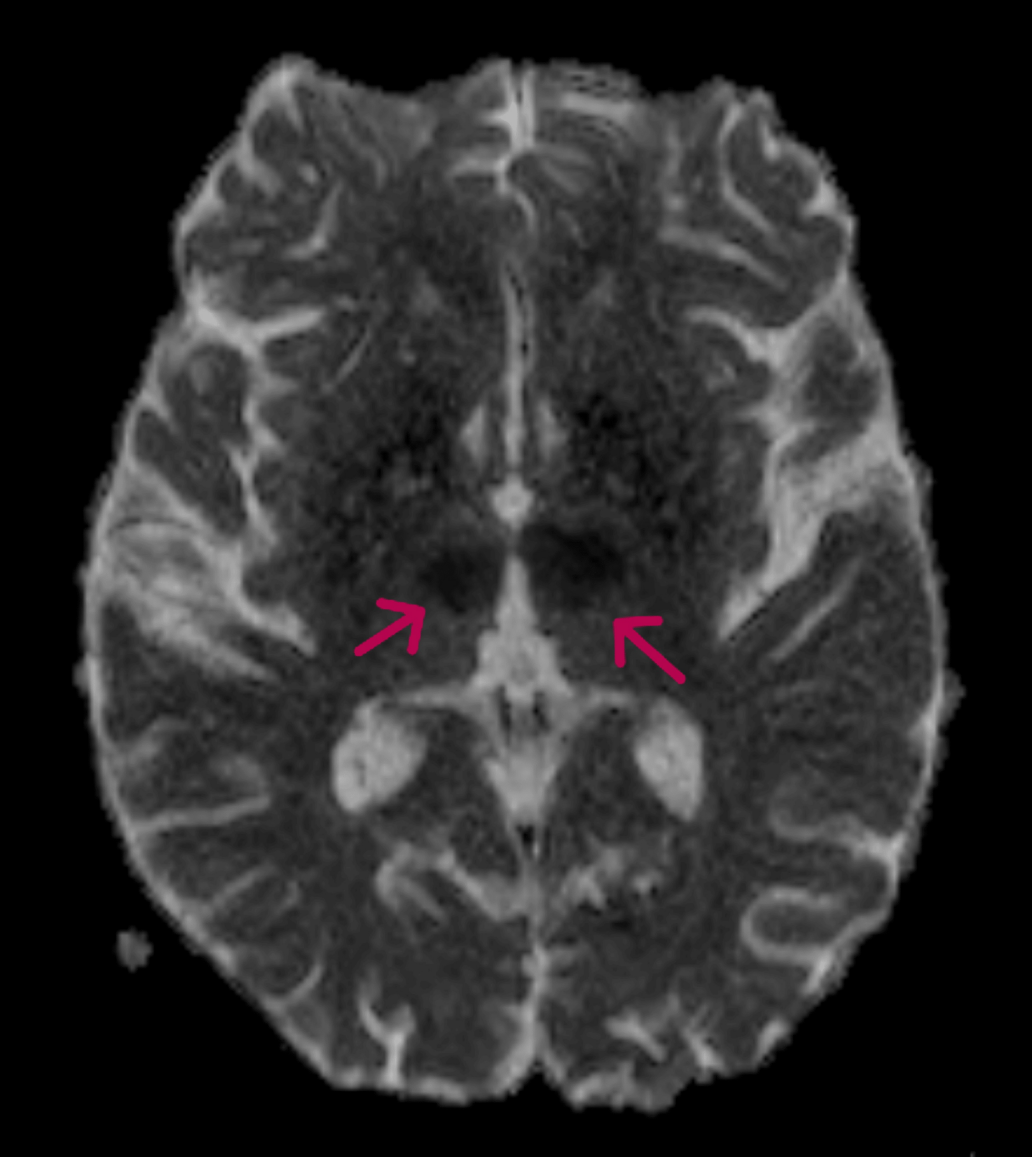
MRI brain (ADC sequence) showing a fall in ADC mapping in the bilateral thalami (arrows). ADC: Apparent diffusion coefficient
